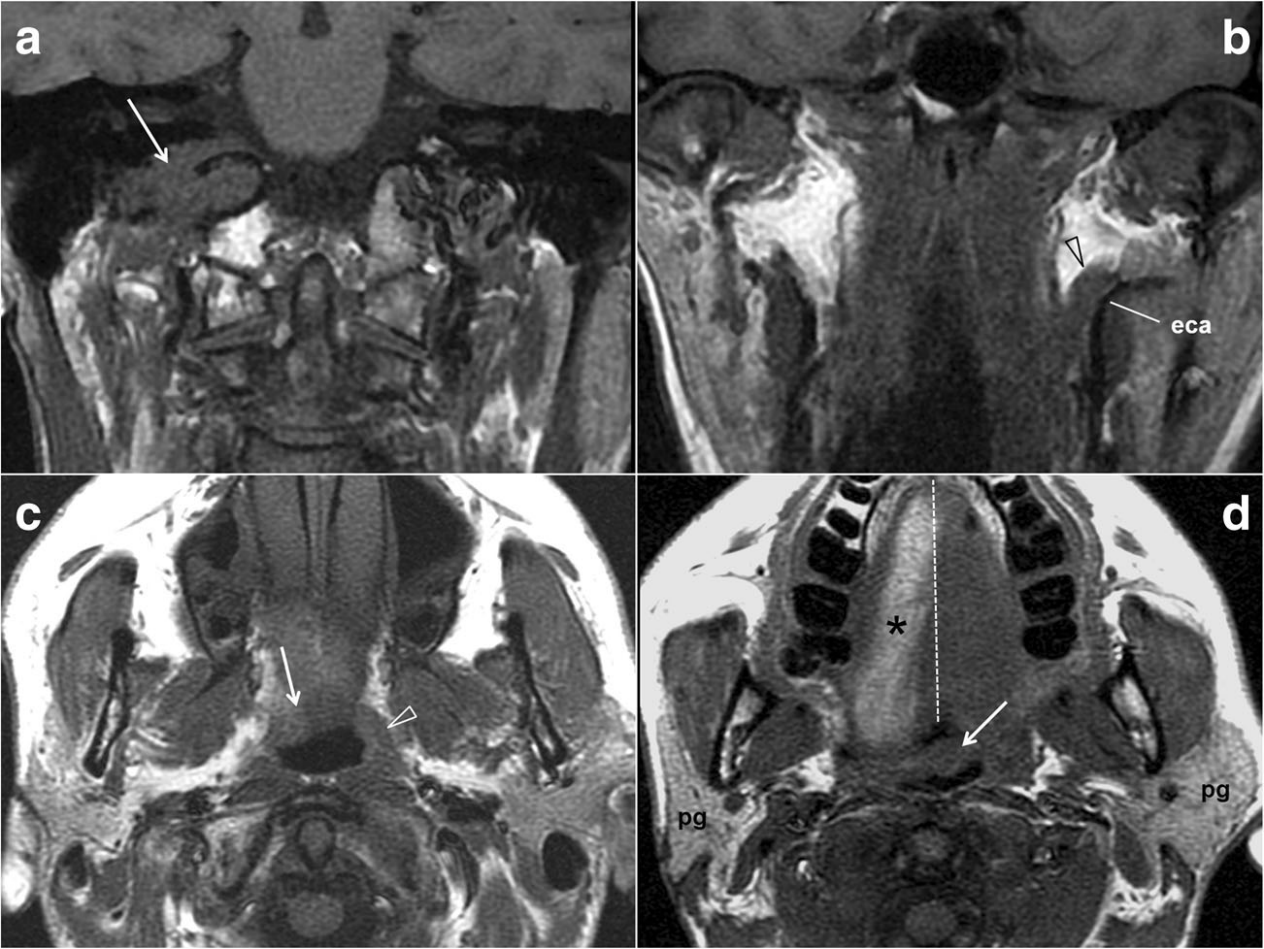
Glossopharyngeal nerve dysfunction. Low cranial nerves palsy. a Coronal fast spin-echo T1-weighted MRI of the neck. A slightly hypointense mass eroding the right jugular foramen (arrow) was demonstrated to be a glomus jugulare paraganglioma. b Coronal fast spin-echo T1-weighted MRI of the neck. The normal stylopharyngeus muscle (arrowhead) on the left and its relationship with the external carotid artery (eca) help to recognise the muscle atrophy on the right side. Stylopharyngeus muscle atrophy is one of the few specific imaging signs of glossopharyngeal nerve dysfunction. c Axial fast spin-echo T1-weighted MRI of the neck. An asymmetric oropharyngeal lumen due to a descending soft palate on the right (arrow) and constrictor muscle atrophy (the arrowhead points to the normal muscles on the left). These are signs of vagus nerve dysfunction, which is normally associated with glossopharyngeal nerve changes. d Axial fast spin-echo T1-weighted MRI of the neck. The uvula (arrow) is displaced to the left side (the dotted midline has been drawn to emphasise that displacement), which is the other typical sign of vagus nerve palsy. Intriguingly, this patient also showed a right parotid gland (pg) atrophy, which might be related to the glossopharyngeal nerve dysfunction. Also note the fat replacement of the right side of the tongue due to hypoglossal nerve palsy
